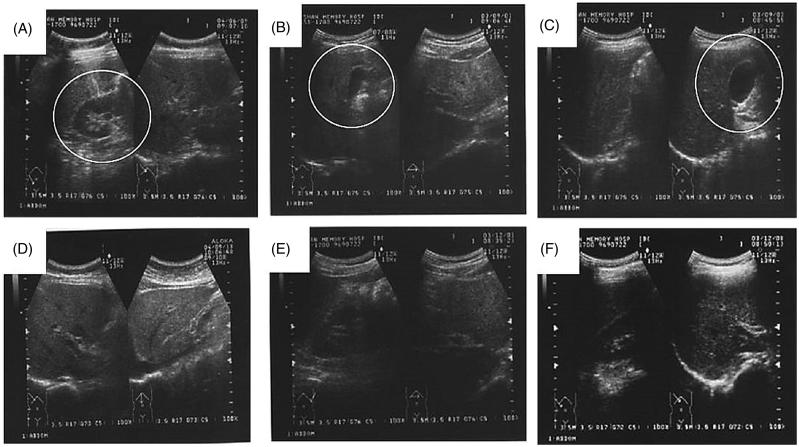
The abdominal ultrasonic image of GL-treated healthy subjects. Image A, B and C represents subject no. 10, 19 at baseline with mild fatty liver and subject no. 36 at baseline with gall bladder polyp (indicated with a circle). Image D, E and F represents subject no. 10, 19 and 36 after 6 months of GL treatment display normal hepatic structure without any signs of fatty liver or gallbladder polyp.
